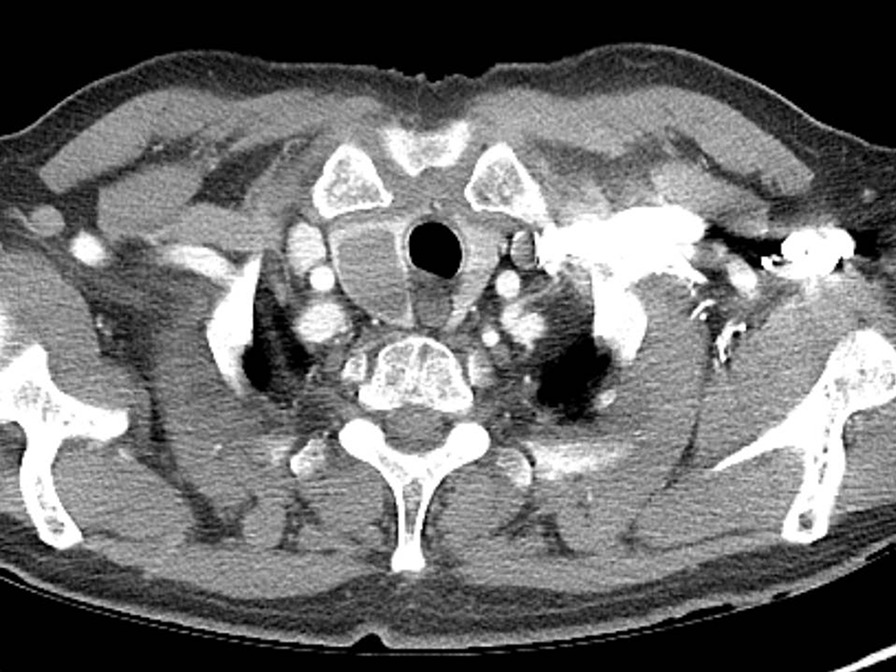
Contrast-enhanced chest computed tomography (CT) taken before ultrasound-guided fine needle aspiration (FNA) of the thyroid gland reveals well-defined low density nodule in right lobe of thyroid gland
